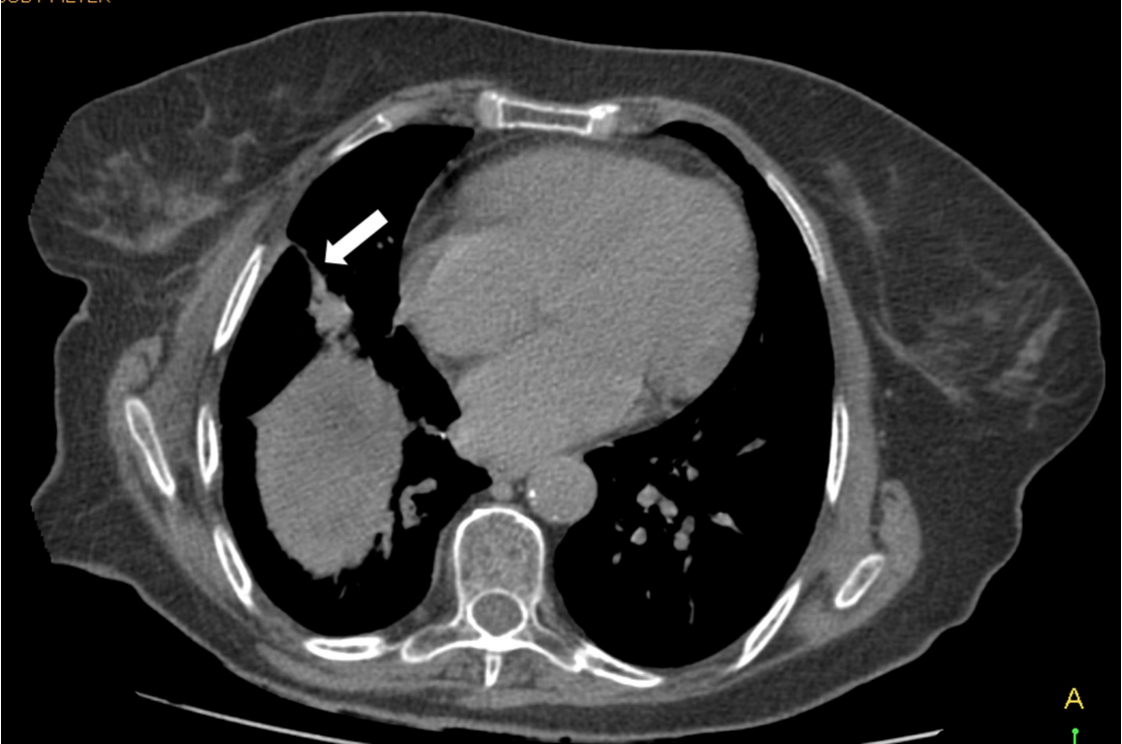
CT scan 2 months postoperatively showing the healed and closed fistulous tract (white arrow).
